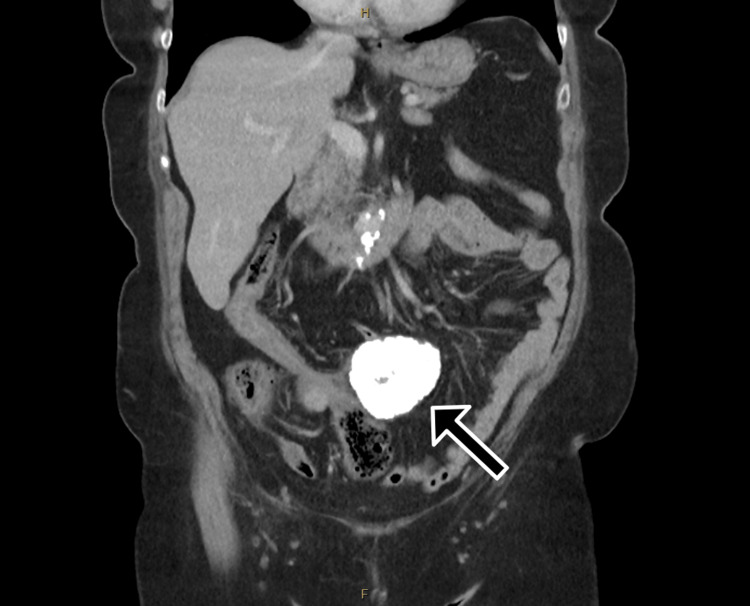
Coronal portal venous phase CT image demonstrating a large calcified mass (arrow) within the central abdomen, with proximal colonic dilatation and distal large bowel collapse consistent with mechanical large bowel obstruction CT: computed tomography
